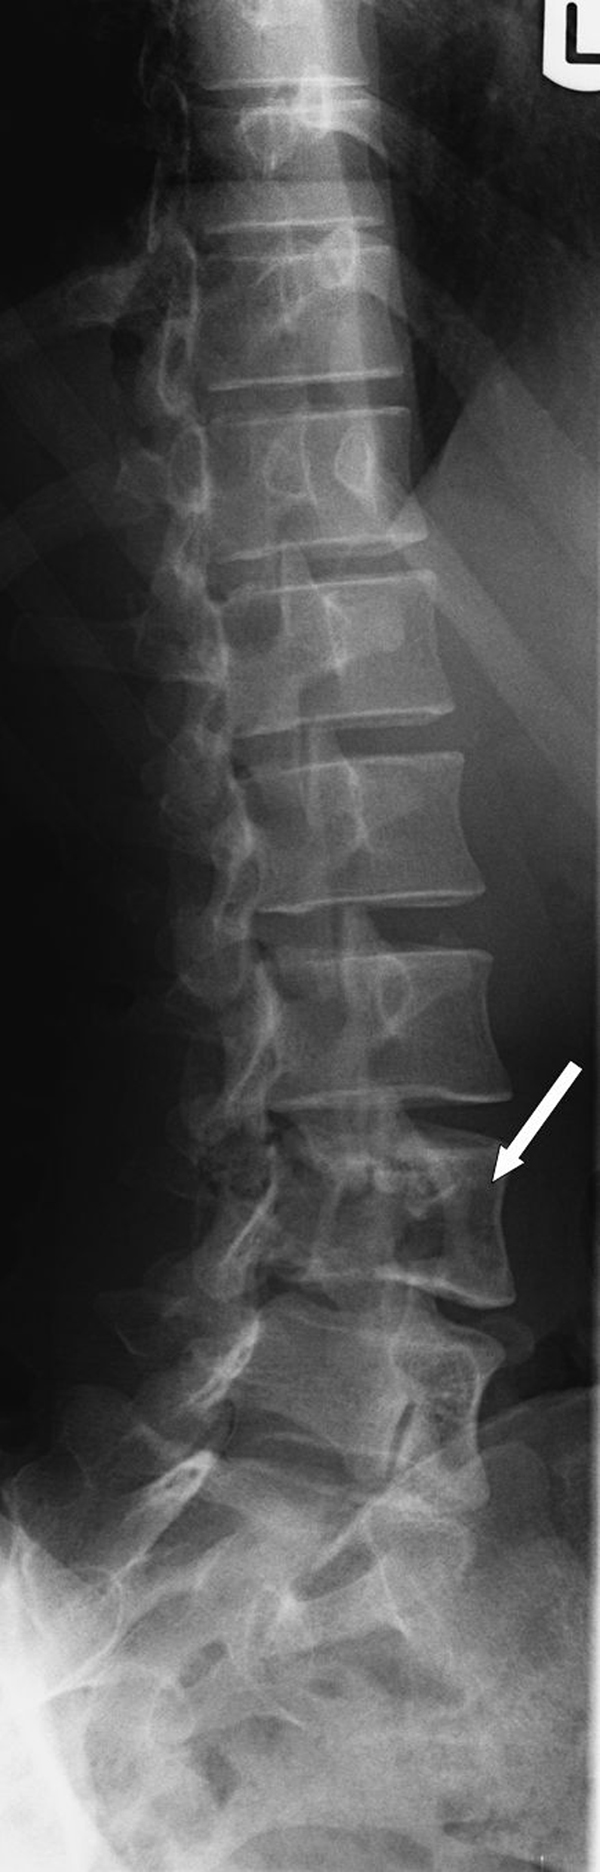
Left oblique radiograph of the lumbar spine demonstrating radiolucency of vertebra L4.
